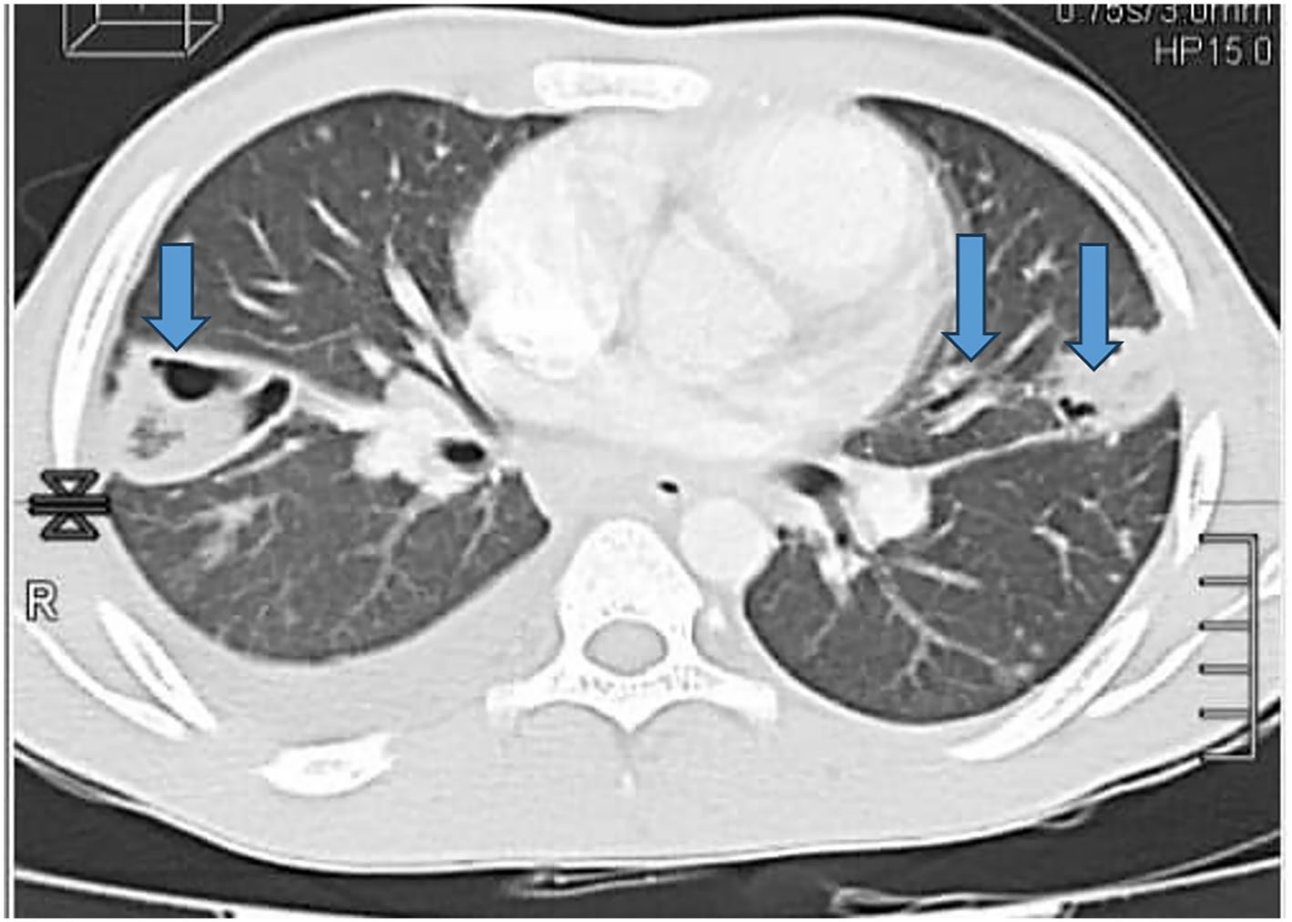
Chest CT scan showing punctate lesions consistent with septic emboli from the cardiac infection and associated lobar pneumonia (See arrows in blue).
